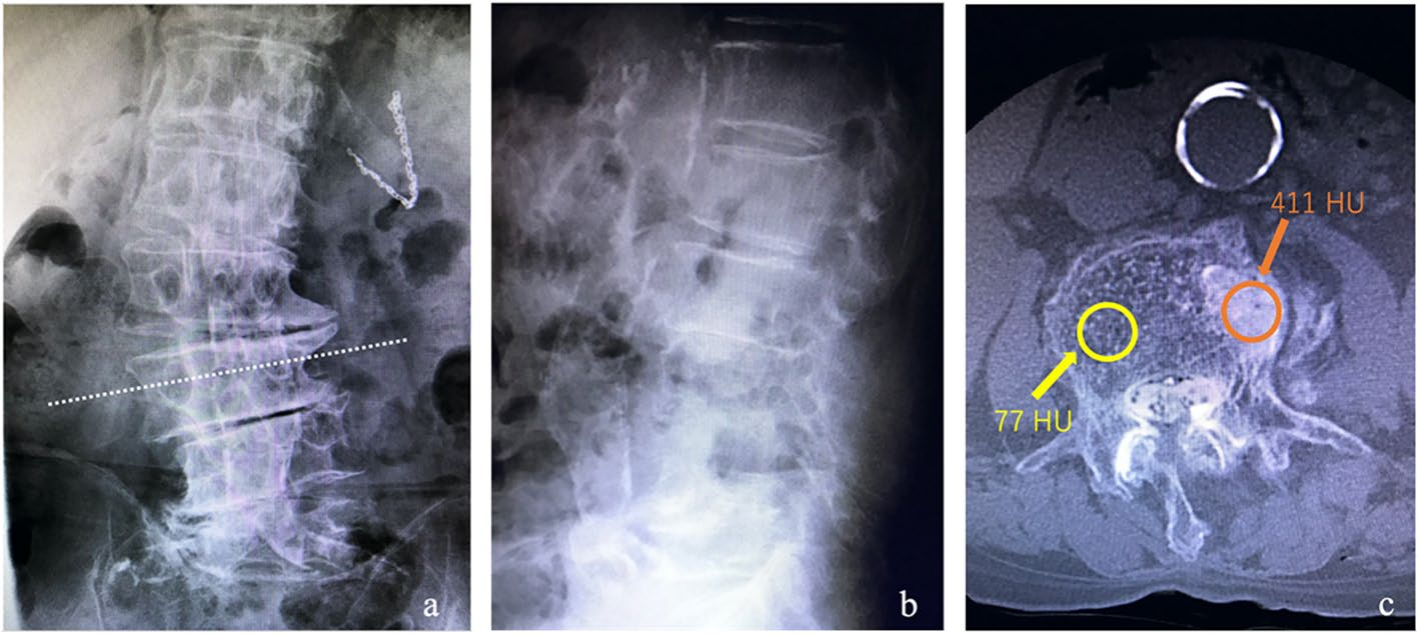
a An anteroposterior radiograph. b A lateral radiograph. c An axial computed tomography image of the fourth lumbar vertebra reconstructed at the level of the dotted line in the anteroposterior radiograph (a)
